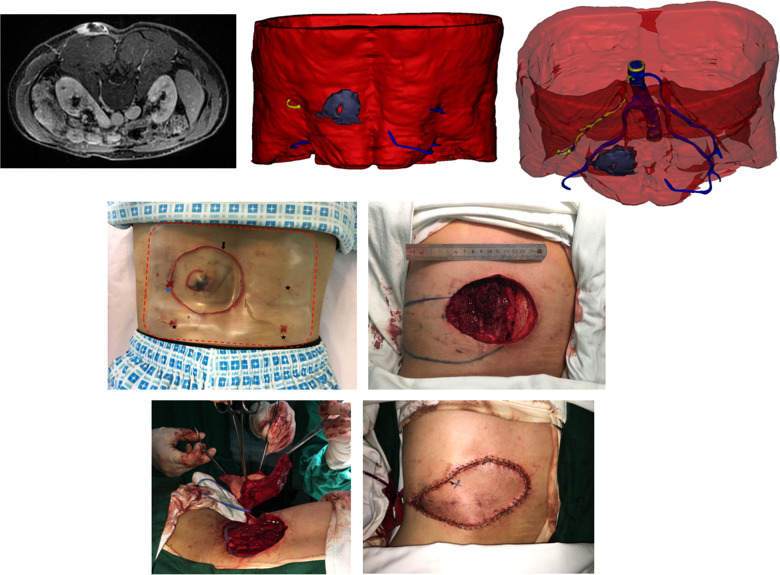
(Above, left) preoperative MRI showing the tumor infiltrating the underlying muscle and adjacent perforator. (Above, middle) 3DR showing the surgical anatomy involving the tumor, underlying muscle, and four perforators. The PPPF was planned based on the yellow perforator. (Above, right) Transparent image showing perforators and main source arteries. (Center, left) Skin incision (black arrow) at 2 cm distance from the tumor boundary and the projection of four perforators according to the surgical guide templates (red dotted line) preoperatively. The blue star represents the perferred yellow perforator, and the three black stars represent the remaining blue perforators. (Center, right) Wound after *en bloc* tumor resection and flap design. The circle represents the piercing site of the superiorly left perforator. The star represents the piercing site of the inferiorly left perforator. (Below, left) harvesting the flap. (Below, right) rotation of the flap for 130° to cover the wound and direct closure of the donor site.
